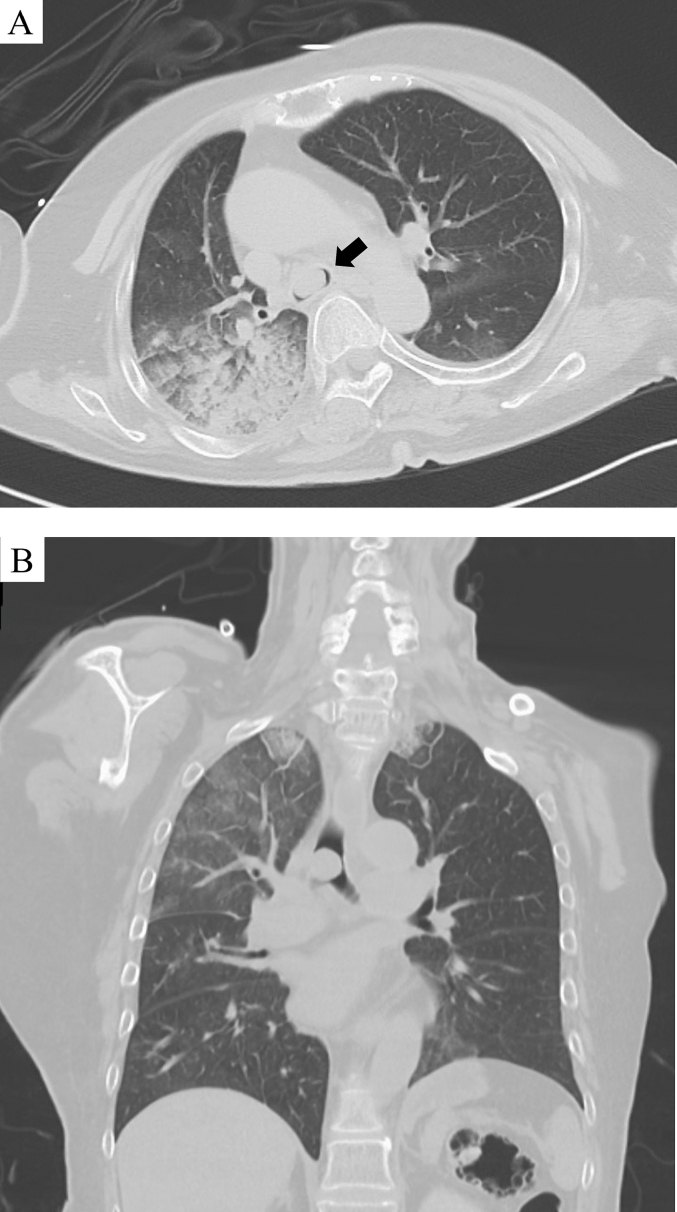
A: Computed tomographic scan of the chest showing a tumor of the trachea and bilateral pneumonia (black arrows); B: Computed tomographic scan with multiplanar reconstruction shows a round lesion obstructing the lumen of the lower trachea located just above the carina.
